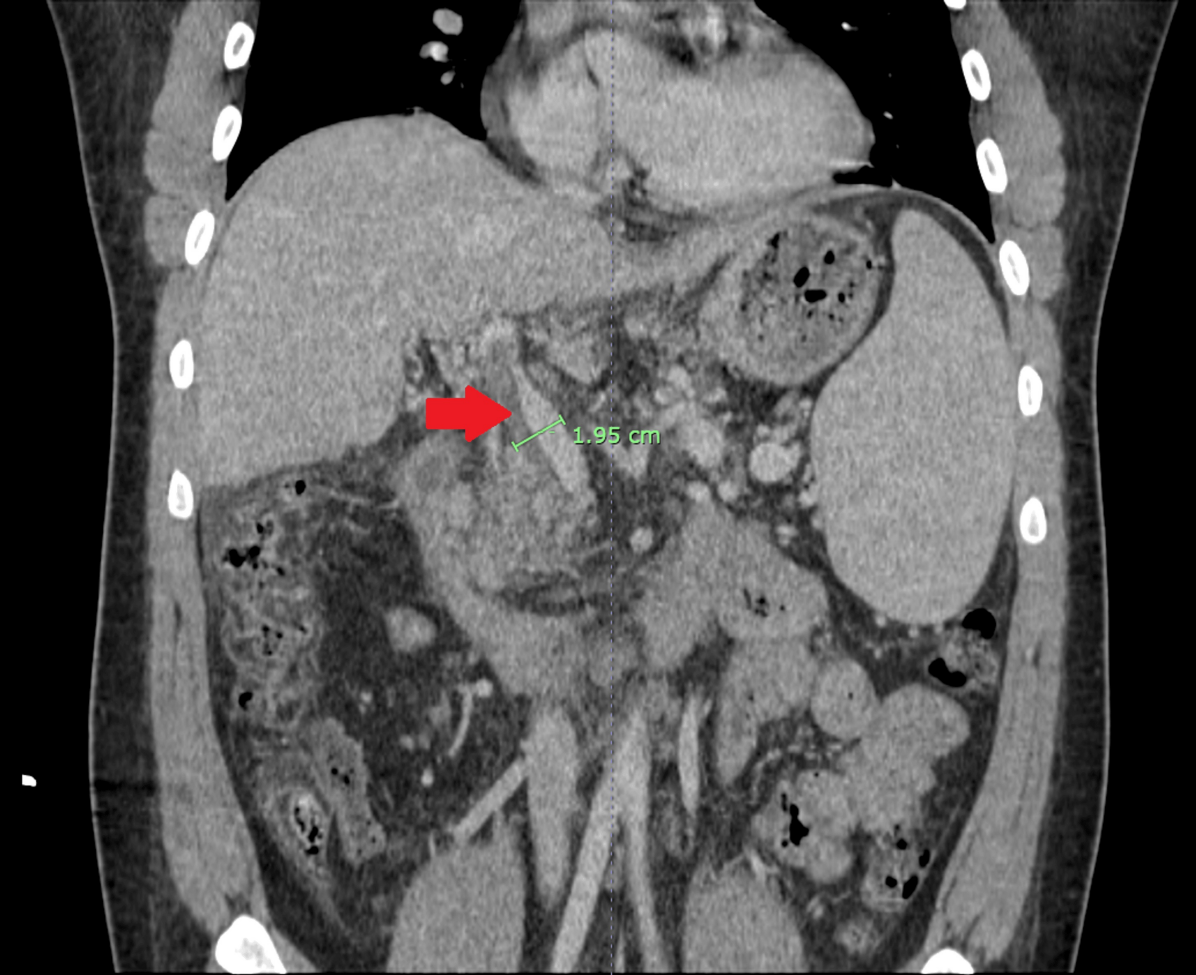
Computed tomography scan abdomen and pelvis Red arrow shows portal vein thrombus and green line shows dilated portal vein consistent with portal hypertension.
